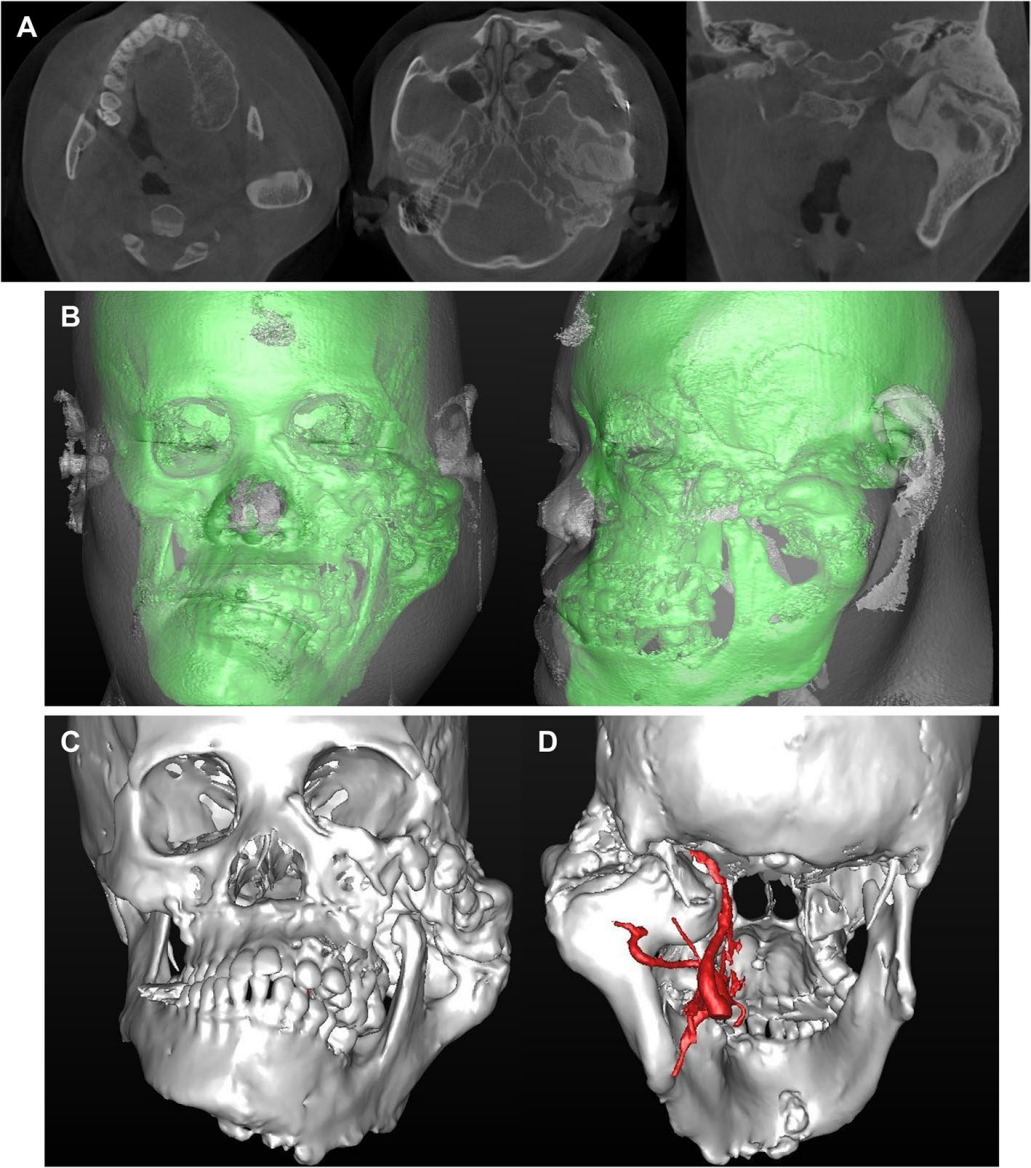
Pretreatment CBCT images and 3D reconstruction. A Axial and coronal CBCT images, B 3D reconstruction of hard and soft tissues, C 3D reconstruction of hard tissue only, D 3D reconstruction of carotid artery using angiography
